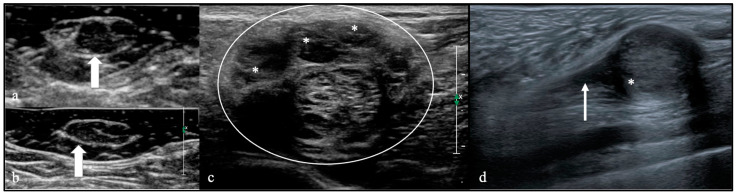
Ultrasound presentation of rare peripheral nerve tumors (PNTs). (a,b) Hypoechoic, enlarged fascicles (white arrow) in a patient with perineurioma, representing Type A in sonotypification. (c) Ultrasound image of a patient with lymphoma manifestation on the sciatic nerve shows a mass with dense, giant fascicles (*) representing Type B. (d) Ultrasound image of a Type C hybrid nerve sheath tumor (schwannoma/perineurioma) originating from the cutaneous antebrachial medial nerve (arrow), with no fascicle structures found in the mass (*).
